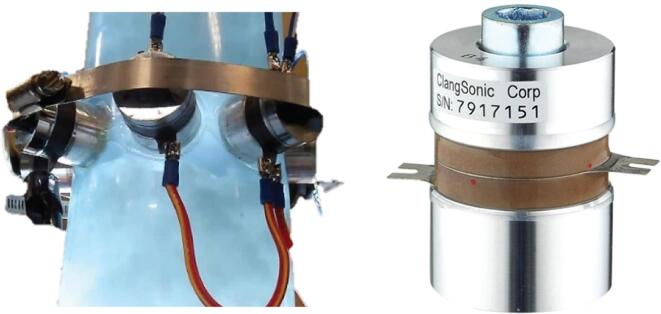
Axisymmetric ultrasonic chamber (left) and used transducers (right).
